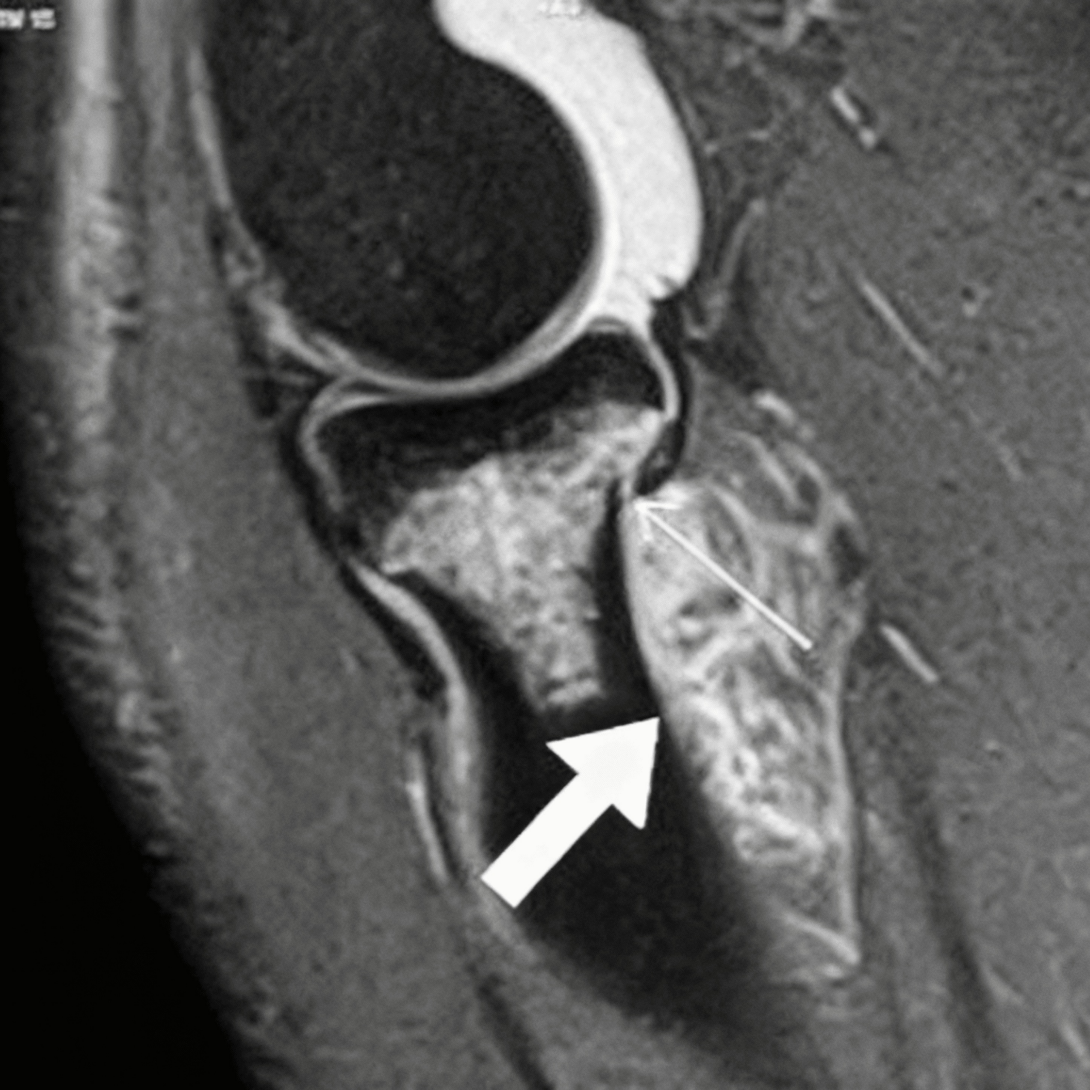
MRI image The thick arrow shows the supinator muscle high-grade tear; the thin arrow shows an undisplaced radial neck fracture.
